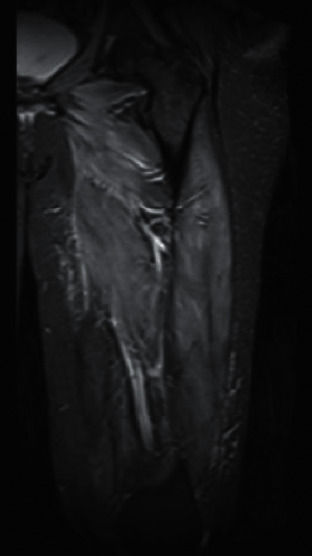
MRI and STIR weighted sequences of the left femur showing extensive intramuscular edema.
